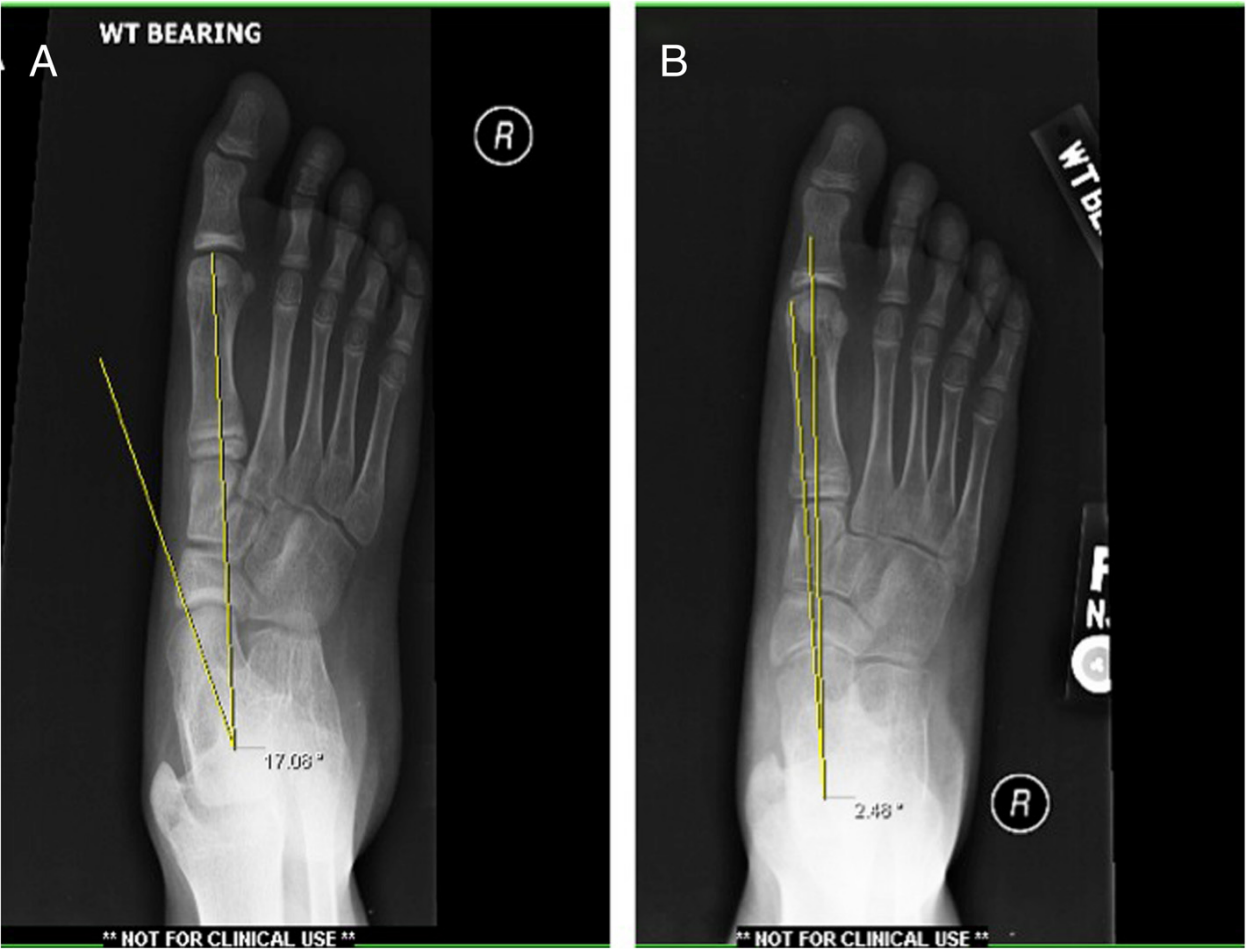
The weight-bearing anteroposterior X-ray shows that the angle of the first tarsometatarsal improves (A and B).
